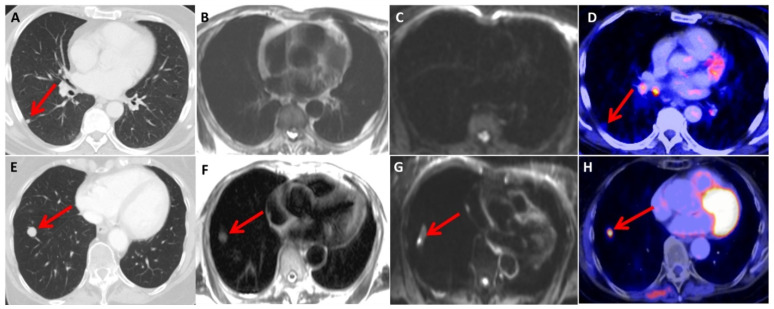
Two melanoma patients (upper row and lower row) presenting with pulmonary metastatic lesions (indicated by the red arrow) in the right lower lobe of the lungs. In the first patient (upper row), the metastasis is visible in the CT scan (A) but not in the HASTE (B) and DWI (C) images. A moderate increase in [18F]FDG uptake is observed in the PET scan (D). The second patient (lower row) had a comparatively larger metastasis, which is visible in the CT scan (E), HASTE (F) image, and DWI (G) image and exhibits intense [18F]FDG accumulation (H).
